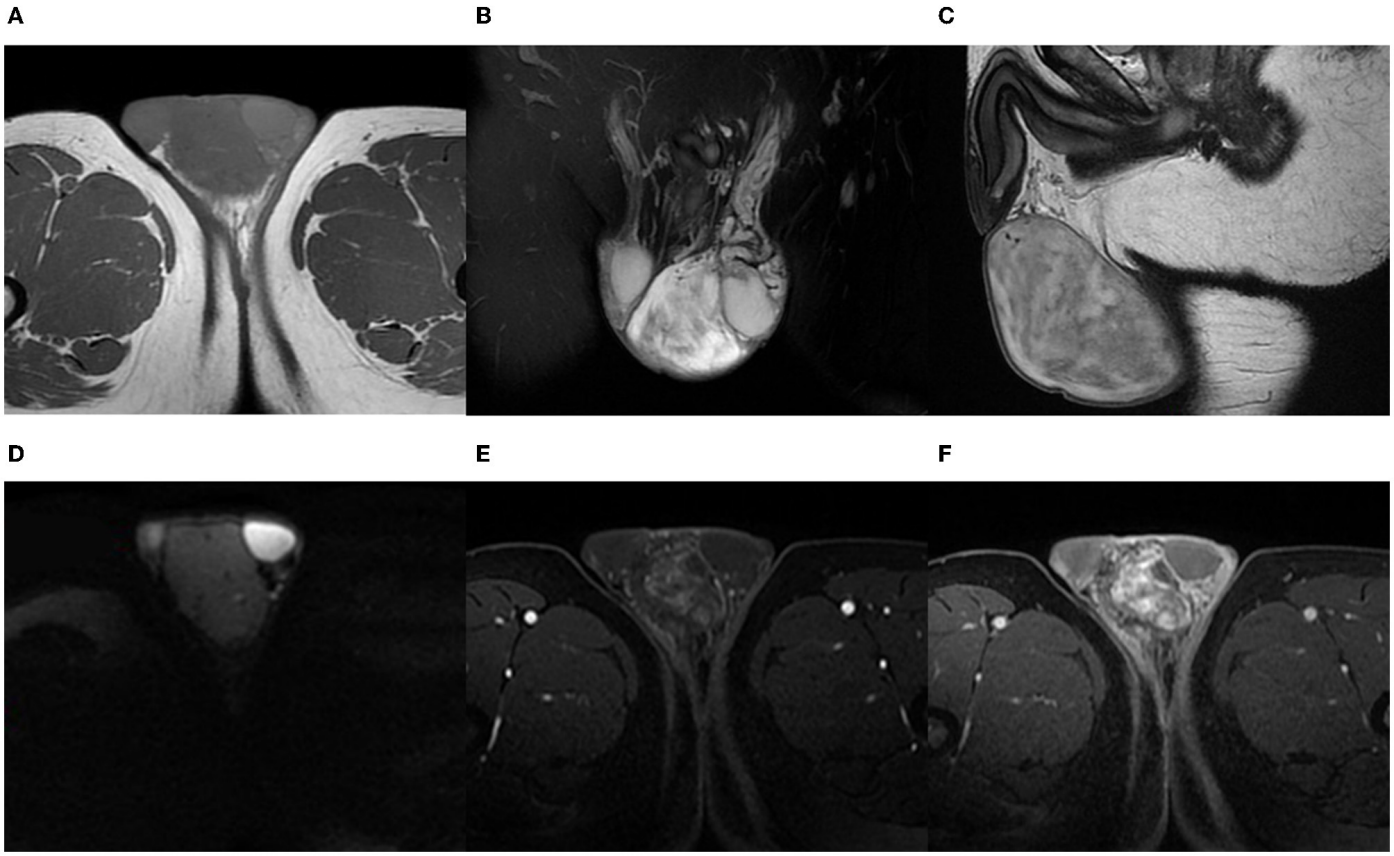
A 42-year-old man with AMF. On T1-weighted images, the tumor reveals low signal intensity but high signal intensity in some circuitous strips (A). On coronal fat-saturated T2-weighted images and sagittal T2-weighted images, the tumor is well-marginated with high inhomogeneous signal intensity (B,C). DWI shows a low-signal tumor (D). The images of contrast-enhanced MRI show a heterogeneous and distinctly enhanced mass. As time goes on, the enhancement of the tumor is more obvious (E,F).
